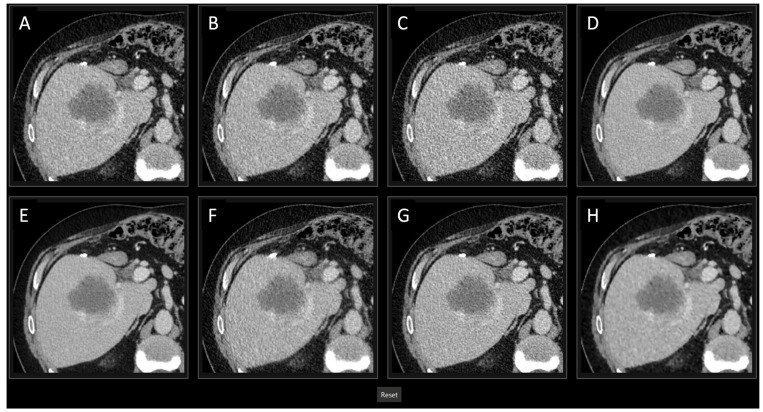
Screenshot of the survey design for the first part of the assessment, with the same region of interest for each reconstruction method. In the first survey, radiologists rated the overall image quality on a scale from 1 (best) to 8 (worst) for various reconstruction methods. The sequence of cases and the arrangement of reconstructions were randomized for each participant and during the evaluation, windowing and zooming were able to be applied. In this case (portal venous), ASIR-V 50% (A), PS Soft (B), FBP (C), DLIR-Medium (D), DLIR-High (E), PS Ultrasoft (F), DLIR-Low (G), and ASIR-V 100% (H).
